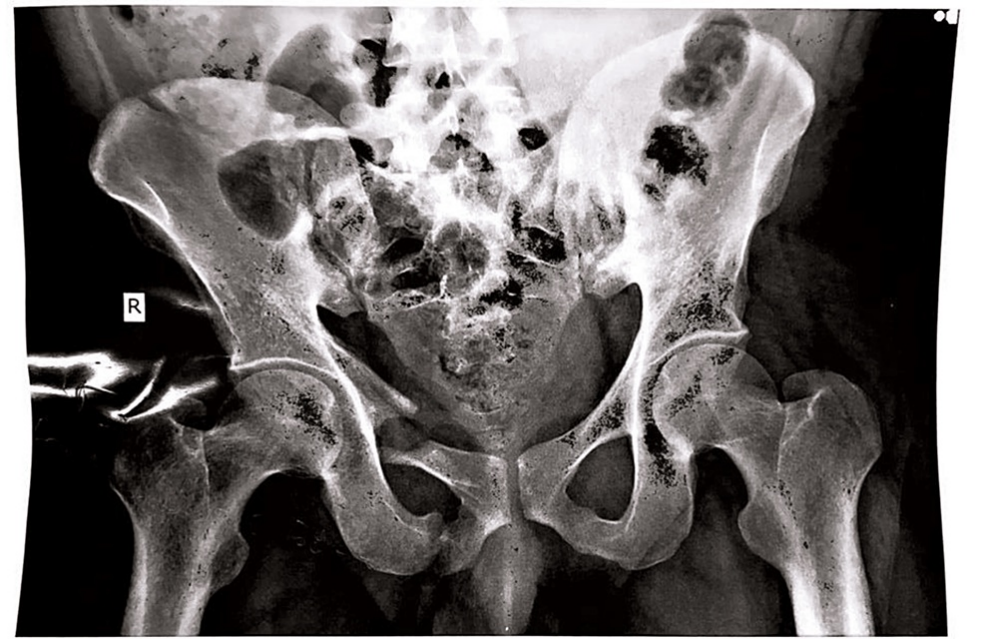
X-ray of the patient's hip
